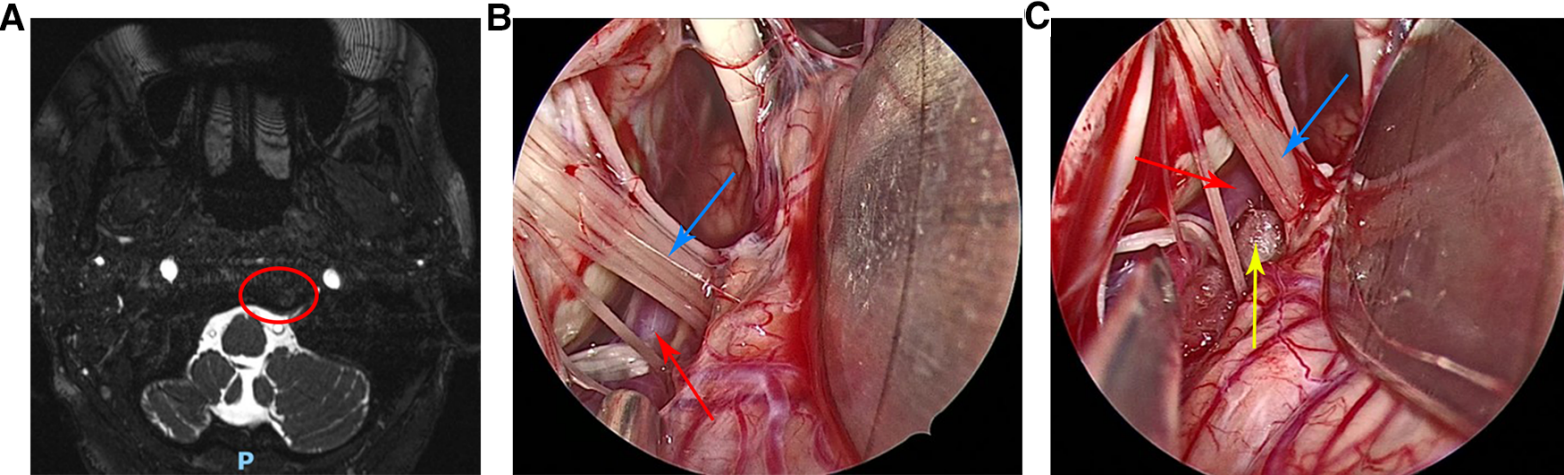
(A) Preoperative head magnetic resonance imaging of the patient. (B) The left vertebral artery and left glossopharyngeal nerve were clearly visible under the endoscopic view. (C) The Teflon pad was placed between the left glossopharyngeal nerve and the left vertebral artery under the endoscopic view. Red circle indicates posterior cranial nerve was compressed by the left vertebral artery, red arrow indicates the left vertebral artery, blue arrow indicates the left glossopharyngeal nerve and the yellow arrow indicates the Teflon pad.
